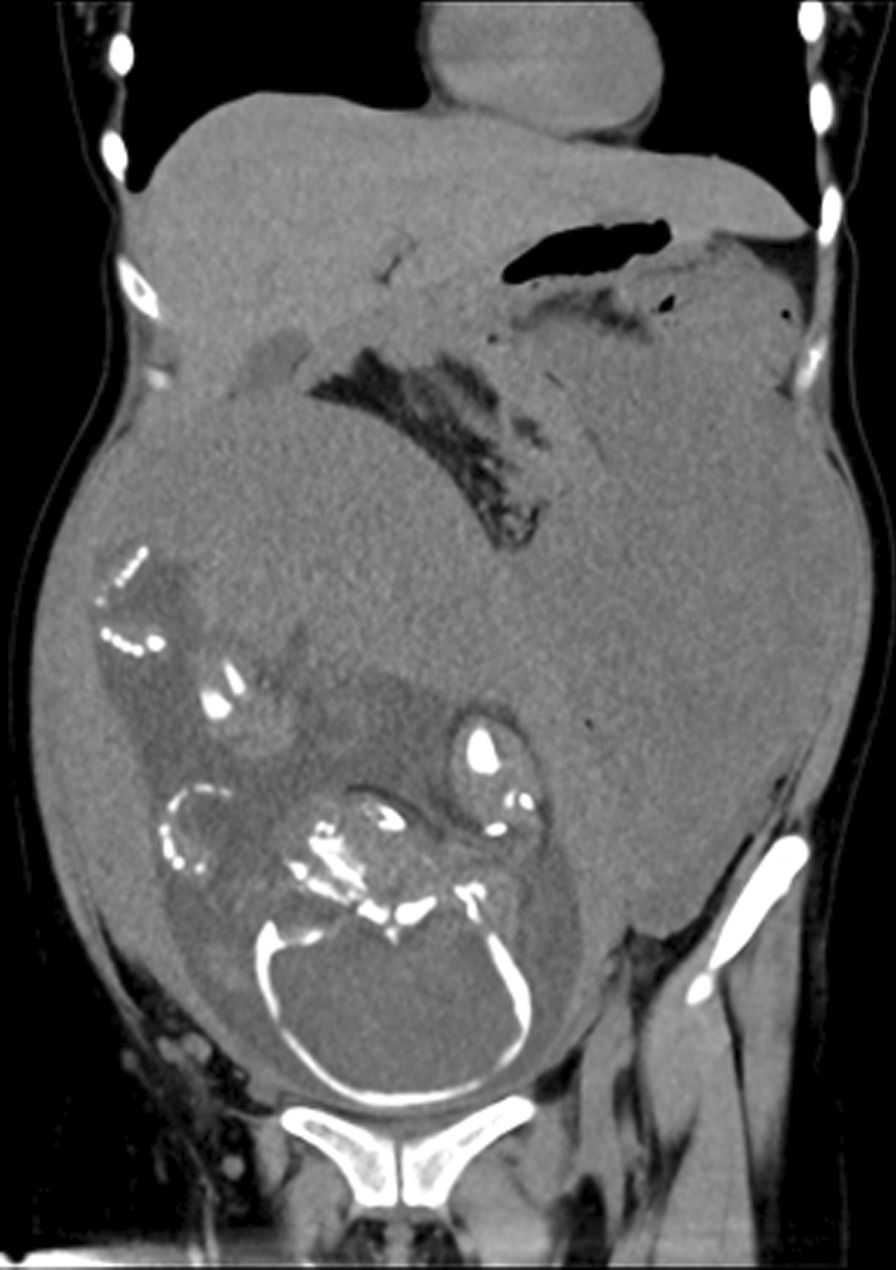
CT scanning showed a huge occupant in left side, abdominal cavity and the fetal in the right with 31 weeks
